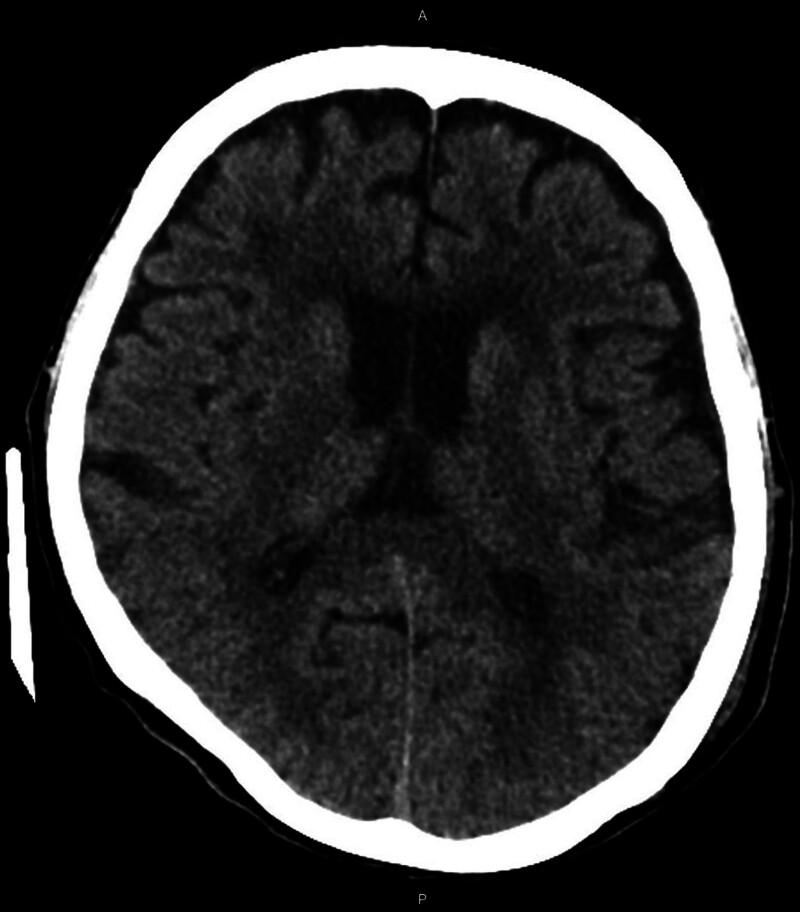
A head computed tomography of this patient.
